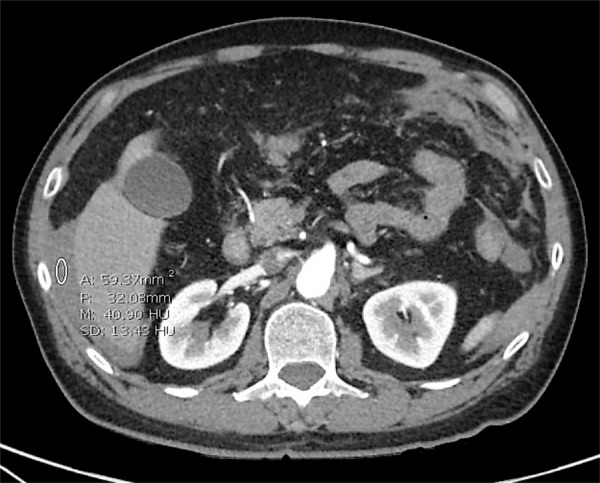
Transverse section on CT abdomen showing free fluid around the liver and subcapsular hepatic cyst CT, computed tomography
